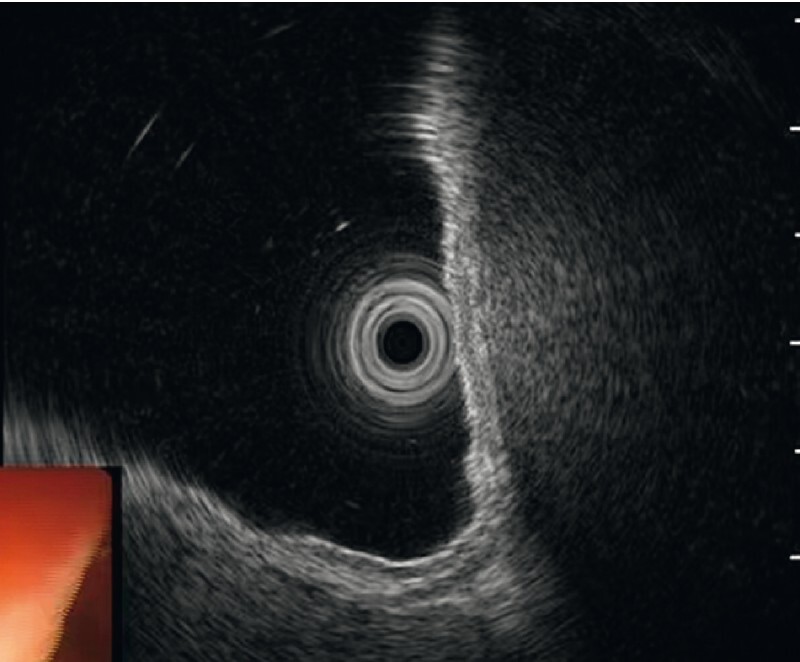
Endoscopic ultrasonography of the gastric fundal protrusion showing that the subepithelial protrusion in the fundus is derived from extraluminal compression.
